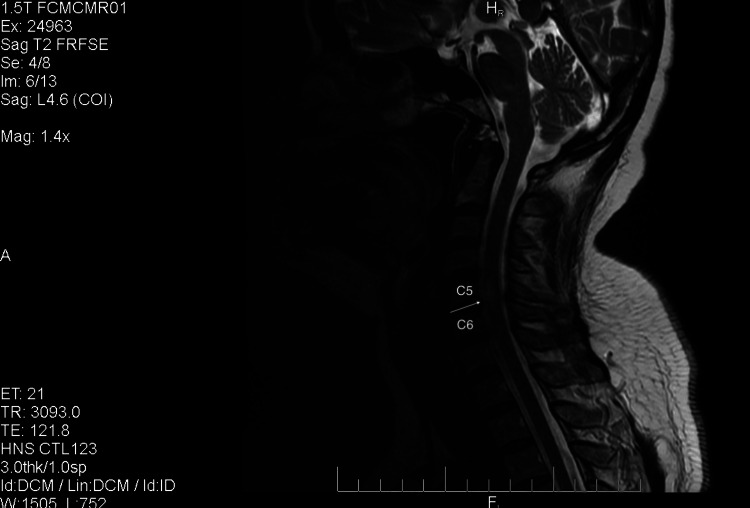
MRI of the patient’s cervical spine, showing T2-hyperintense signal over the cervical spine, consistent with edema at the C5-C6 level.
